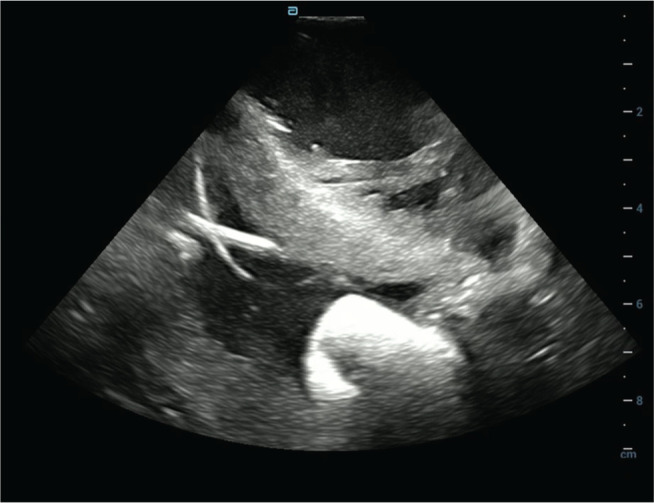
Intracardiac ultrasound showing the HD Grid mapping catheter via a transseptal approach, guiding the Sphere-9™ catheter via a retrograde aortic approach (see Video 1).
